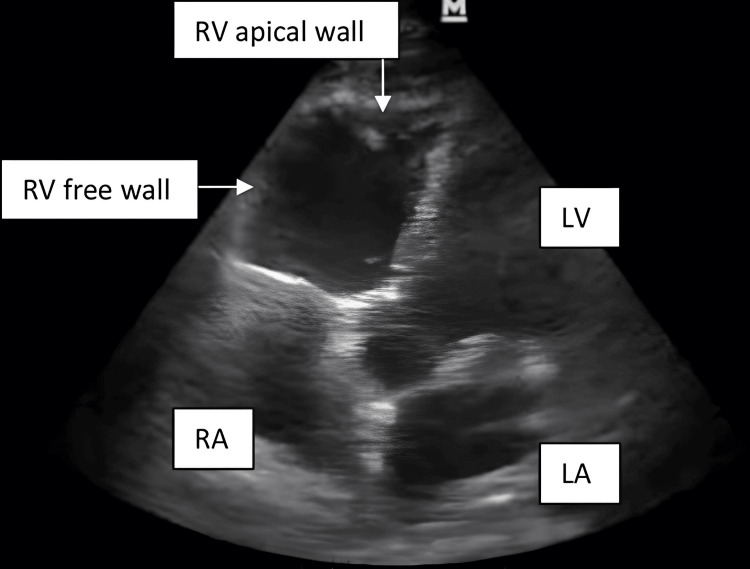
Hypokinetic and severely dilated RV in the setting of acute RV failure secondary to BCIS visualized intraoperatively on TTE via apical 5 chamber view. RV, right ventricle; BCIS, bone cement implantation syndrome; TTE, transthoracic echocardiography; RA, right atrium; LV, left ventricular; LA, left atrium
